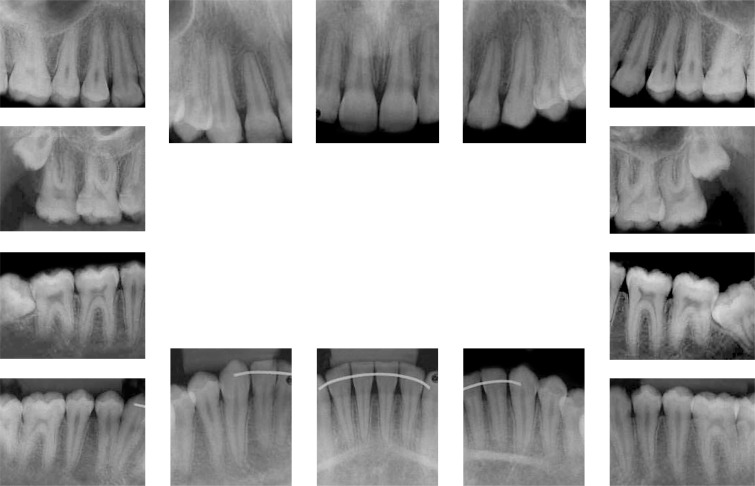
Final periapical radiographs.
